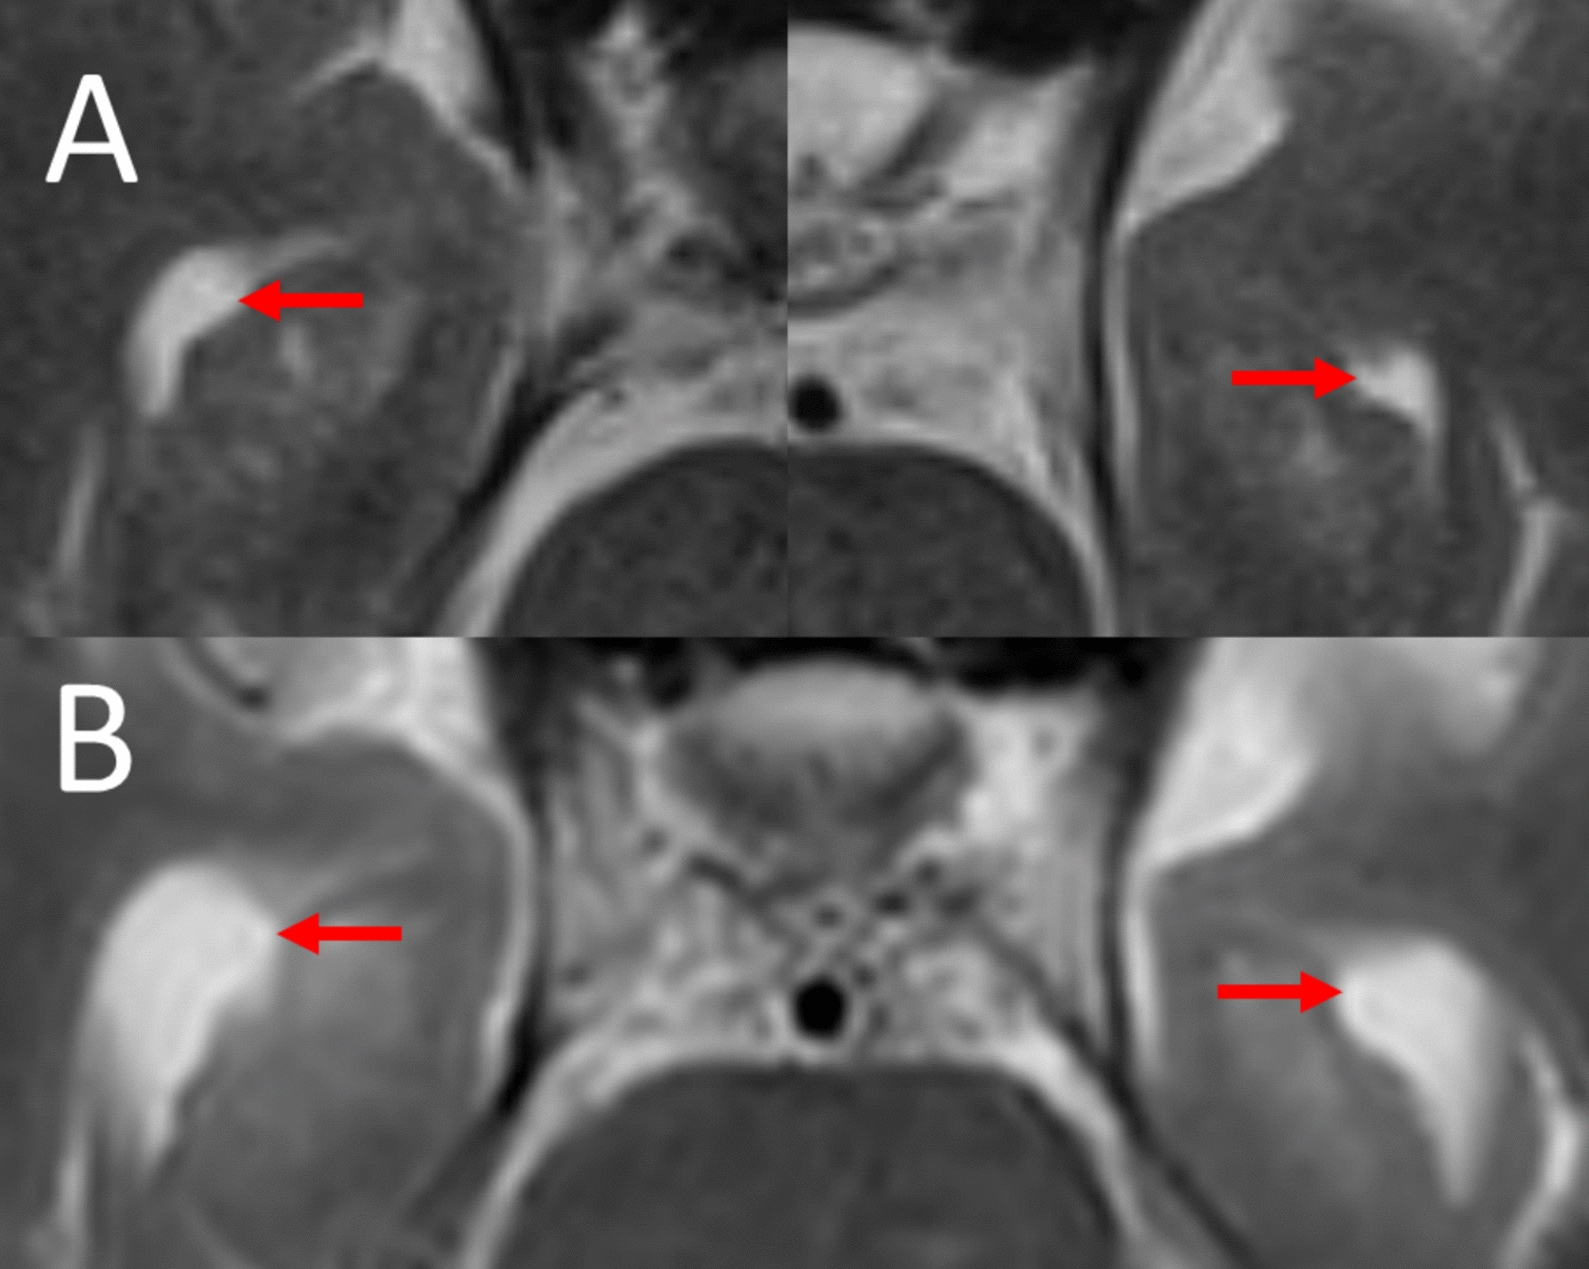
Hydrocephalus internus caused by an infratentorial dorsal foramen magnum meningioma after 7 years (transverse-axial T2-weighted magnetic resonance imaging sections). A Normal width of the temporal horns of the lateral ventricles (arrows). B Increase in the width of the temporal horns of the lateral ventricles in the follow-up scan indicative of obstructive hydrocephalus (arrows)
